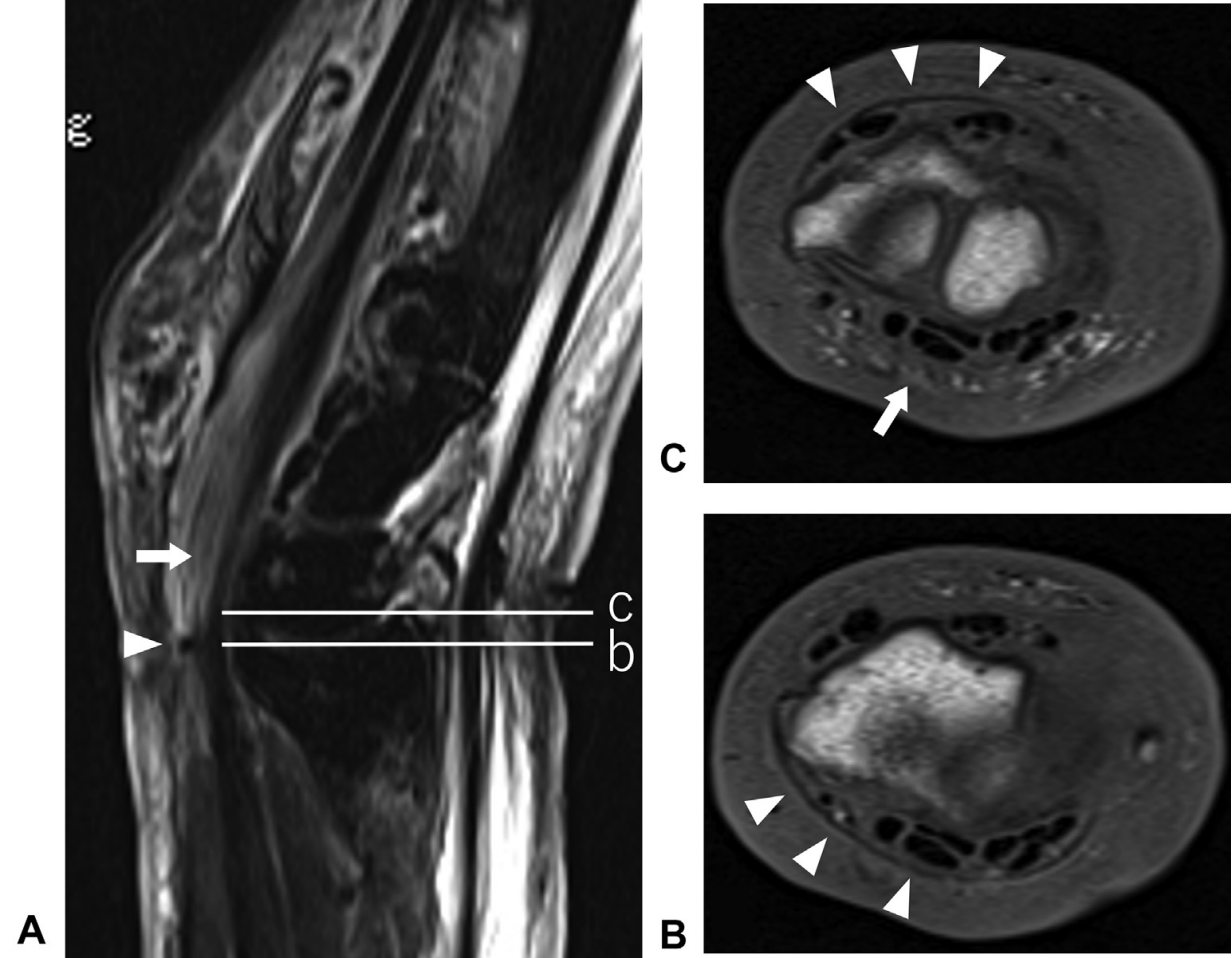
Preoperative MRI. A Sagittal T2-weighted imaging showing edematous changes in the whole hand and a cord-like object (arrowhead) compressing the median nerve (arrow). B Axial T1-weighted imaging at the level of nerve entrapment showing a cord-like object on the volar side (arrowheads); the median nerve is not visible due to entrapment. C Axial T1-weighted imaging at 1 slice distal from image B, showing a cord-like object on the dorsal side (arrowheads), with the median nerve visible on the volar side (arrow).
